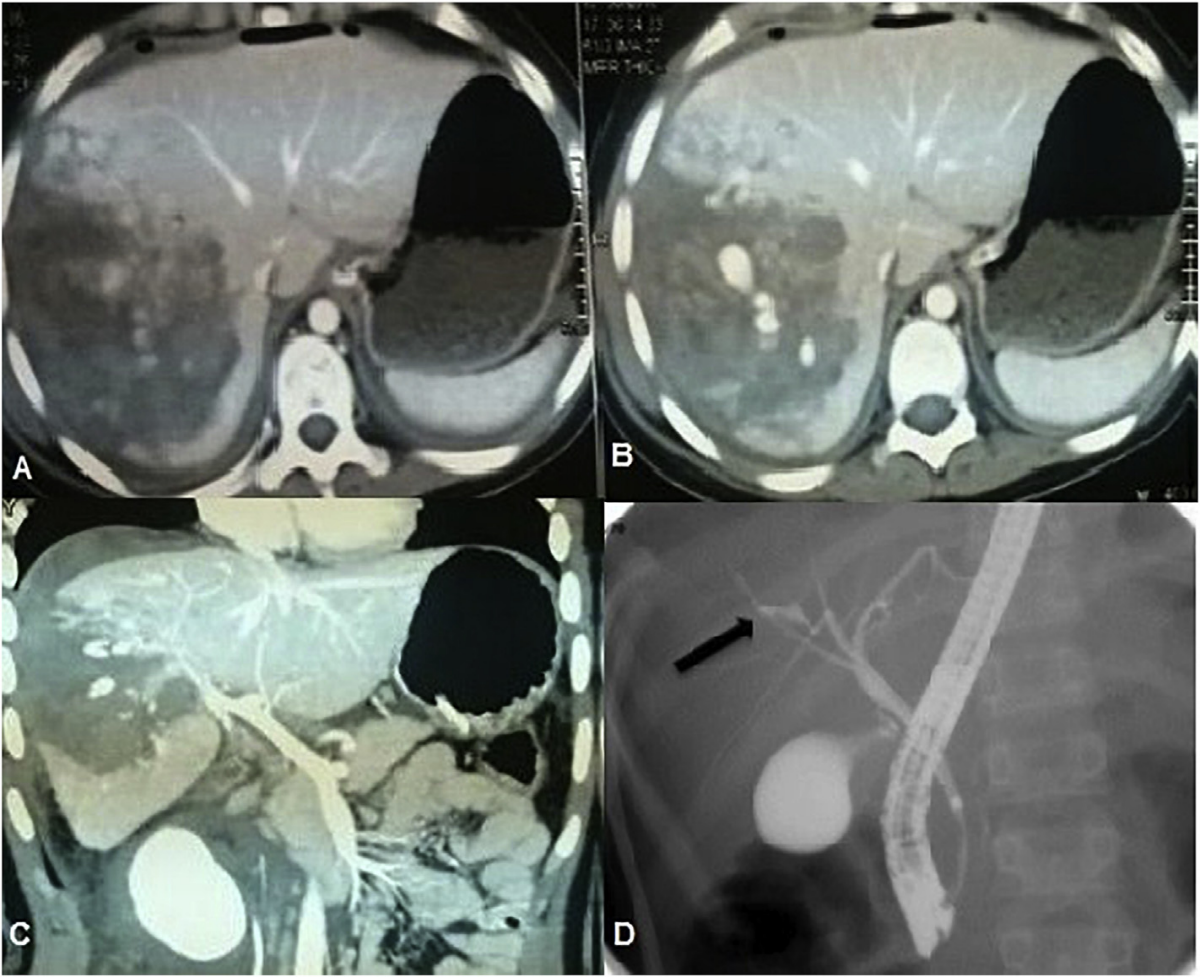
A,B,C) CT pictures of female patient 12 years old with RTA blunt abdominal trauma grade V, she had lacerated right hepatic lobe with large hematoma involving segments 6,7,8, intraparenchymal extravasation of the dye is seen. D) ERCP demonstrating bile leak (arrow indicates bile leak), of Rt anterior duct.
